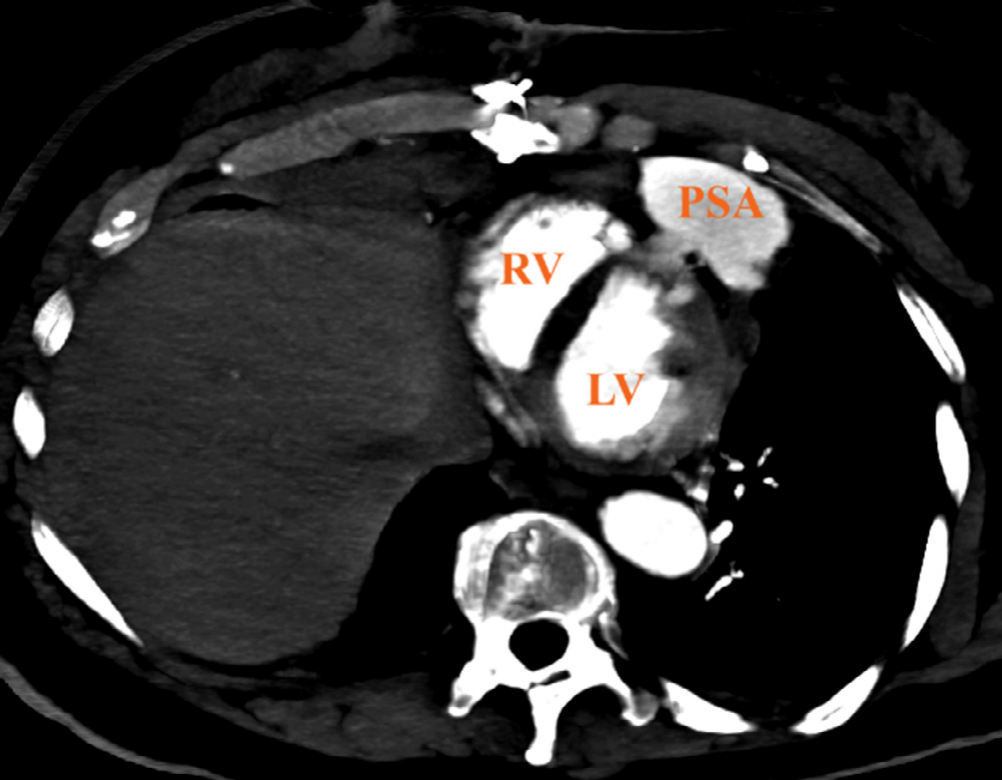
Axial MIP Chest CT identifying the communicating defect of the contained pseudoaneurysm (PSA) involving the right ventricle (RV) and left ventricle (LV). The violation to the right ventricle measured roughly 0.7 × 0.5 × 0.2 cm and an estimated 0.7 × 1.1 × 0.2 cm defect at the left wall. The body of the pseudoaneurysm measured 6.7 × 3.1 × 3.5 cm.
